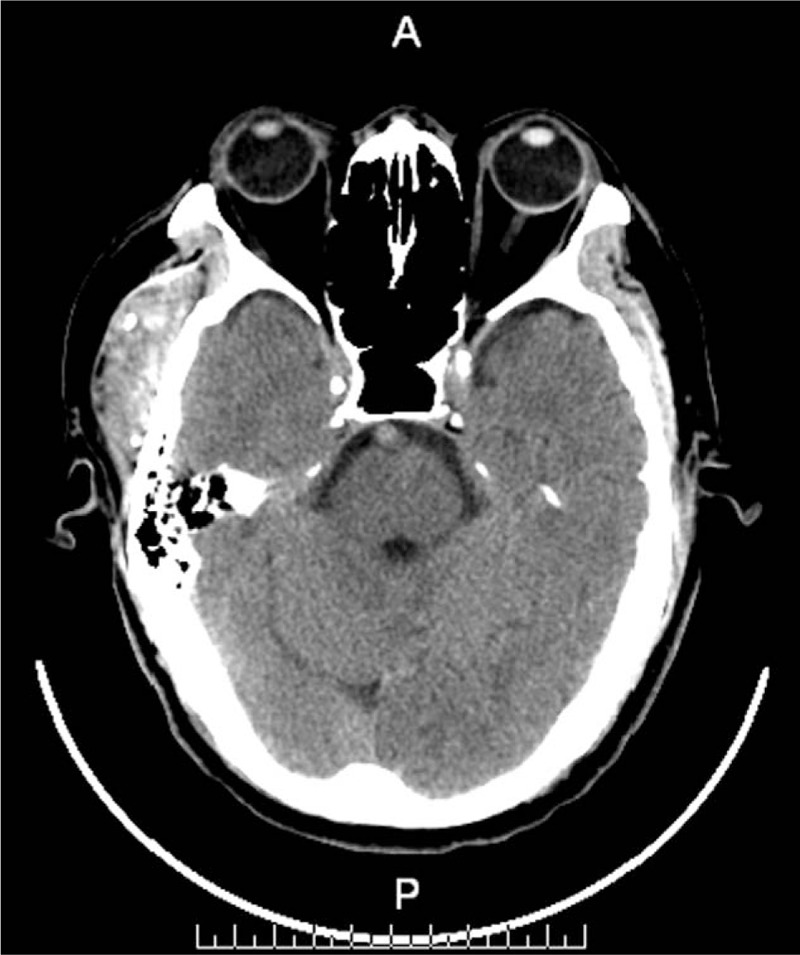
Computed tomography shows the mass in the right temporal region with many calcified nodules and no erosion of the bone.
